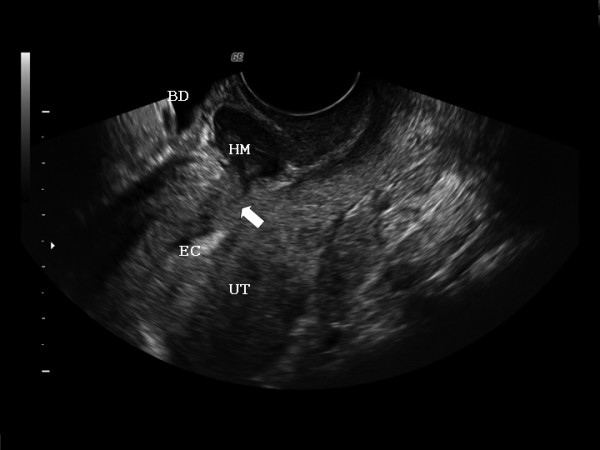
Two-dimensional transvaginal uterine (UT) ultrasound on longitudinal plane showing the communication (arrow) of the hematoma (HM) with the endometrial cavity (EC).
